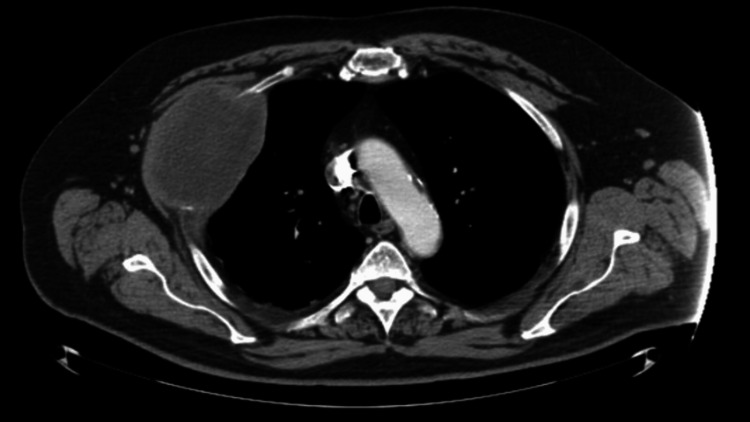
CT of the chest (axial view) showing a well-circumscribed low-attenuation ellipsoid mass measuring approximately 10.3 × 6.6 cm involving the right anterolateral chest wall.
